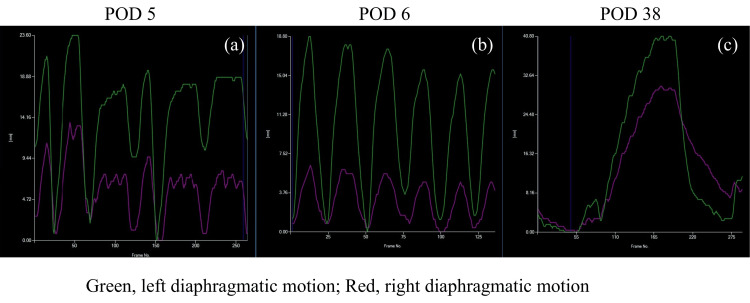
Patient’s diaphragmatic motion assessed using dynamic digital radiography (DDR) (DM-mode) observed, the right diaphragm exhibits a weaker motion than the left side. Vertical axis: Diaphragm displacement distance. Horizontal axis: Time. DM-mode: diaphragm movement mode
